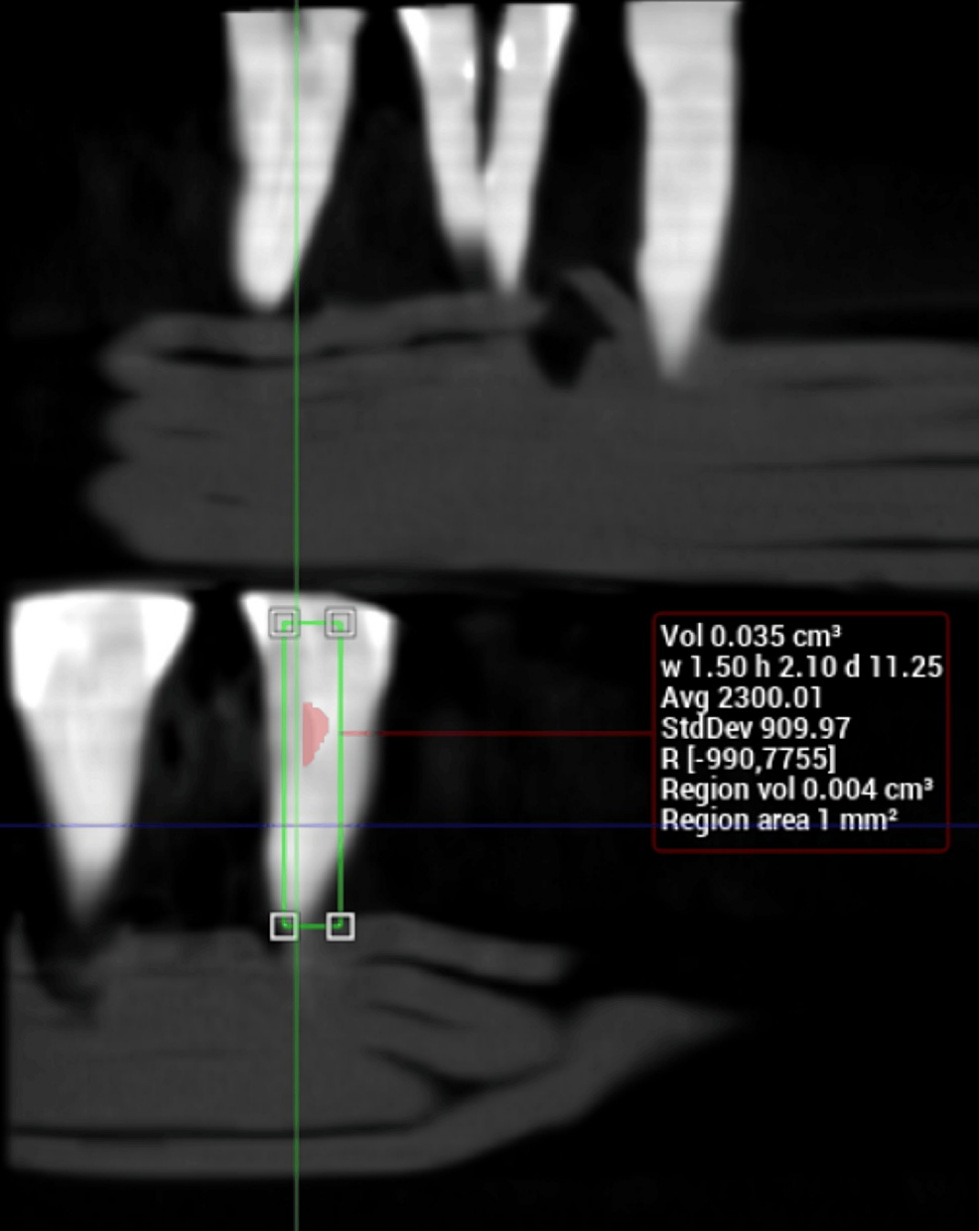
Calculation of volume from CBCT image after retreatment with D-RaCe CBCT: Cone beam computed tomography
